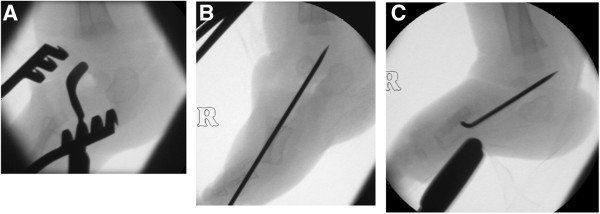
X-ray series showing surgical correction and stabilisation - intraoperative lateral image intensifier views of right foot. (A) A dorsomedial incision has been used to approach the talonavicular joint. Note the obliquity of the bean-shaped ossification centre of the talus. (B) The talonavicular joint has been reduced. Note the new axis of the talar ossification centre. (C) The reduction is maintained as the ankle is dorsiflexed after tendo Achilles lengthening.
